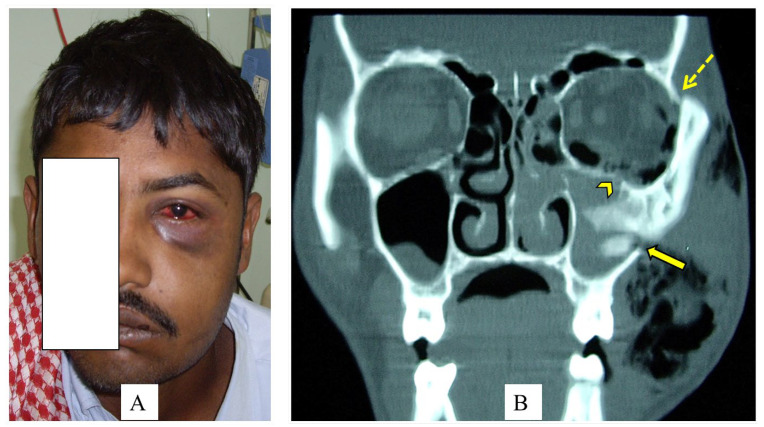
A 28-year-old camel caregiver was kicked in his face by a camel’s front leg (A). The left side of the face is swollen with clinically apparent subcutaneous emphysema, periorbital edema, ecchymosis, and subconjunctival hemorrhage. The coronal view CT scan of the facial bone (B) shows a fracture of the left superolateral orbital rim (hashed arrow), a comminuted fracture through the left orbital floor (arrowhead), and a fracture of the lateral wall of the left maxillary sinus (arrow). Specific consent has been obtained from the patient for the publication of identifying figures/images.
